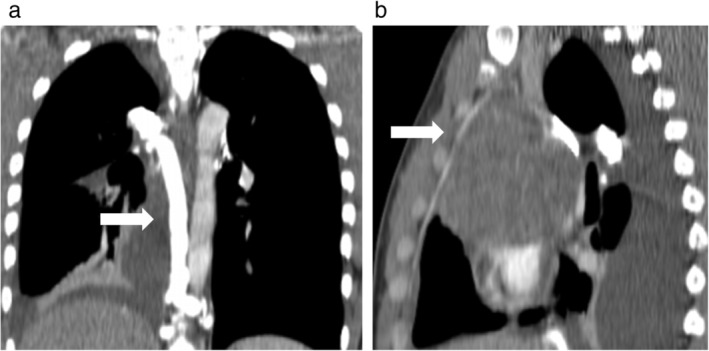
Collateral venous system seen at baseline CT scan. (a) Enlarged azygos vein (arrow) reached the diameter of the adjacent thoracic aorta (coronal view, multiplanar reconstruction). (b) Internal mammary vein (arrow) wasnot affected by collateral overflow (sagittal view, multiplanar reconstruction).
